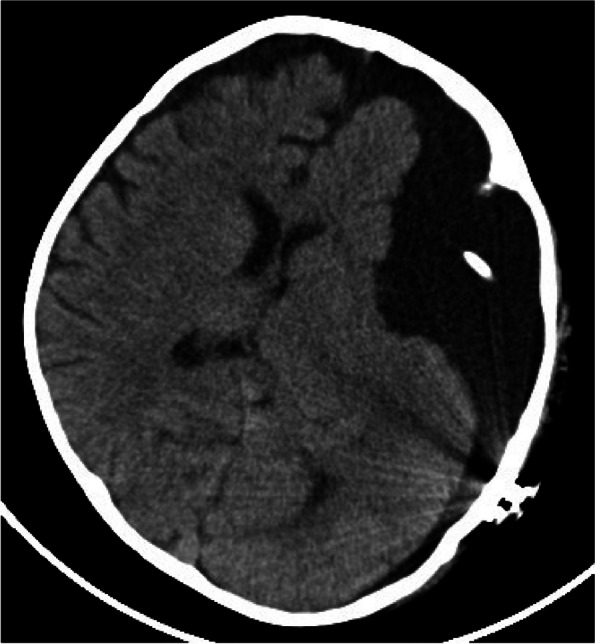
Postoperative cranial CT scan demonstrating a reduction in subdural effusion following cysto-peritoneal shunt placement
